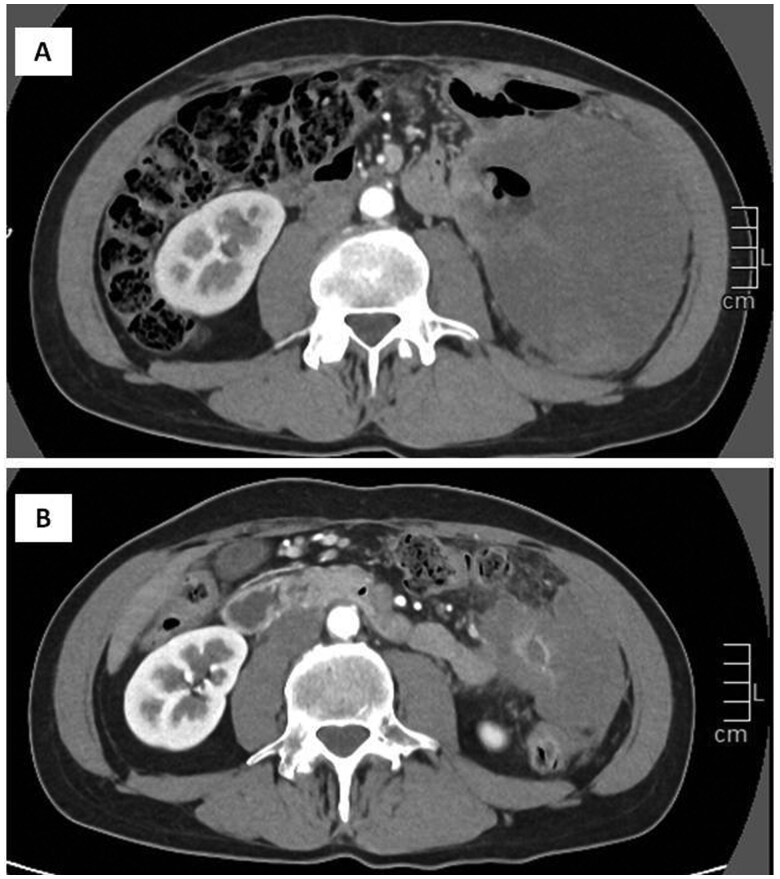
CT scan of jejunal lesion at baseline (A) and after (B) chemo-immunotherapy.
